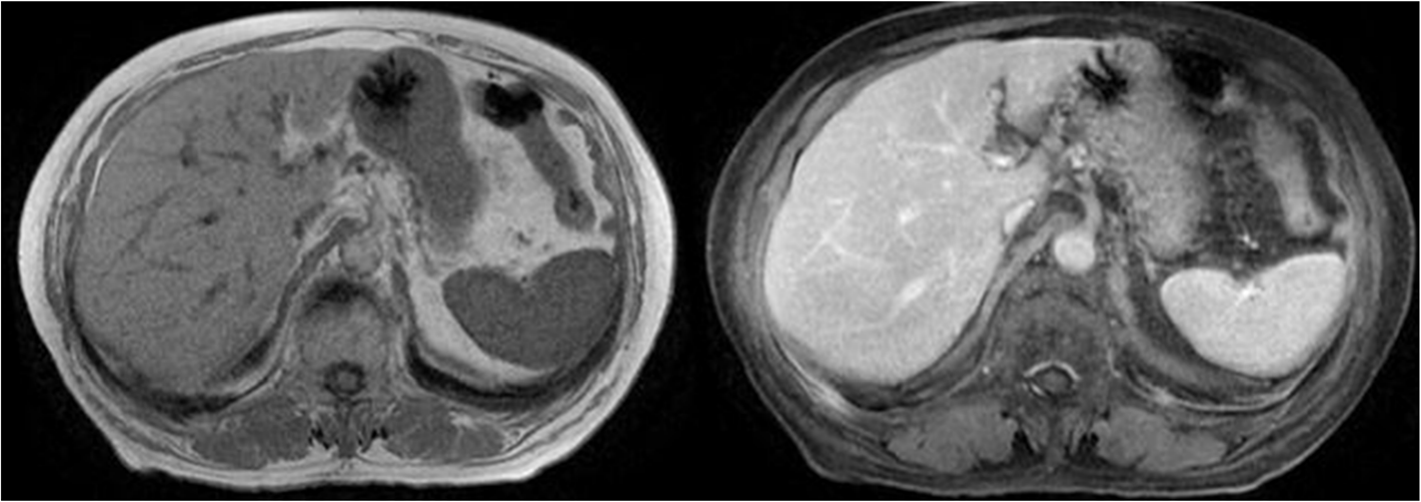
The MRI performed at three weeks showed the initial reduction of the lesions
